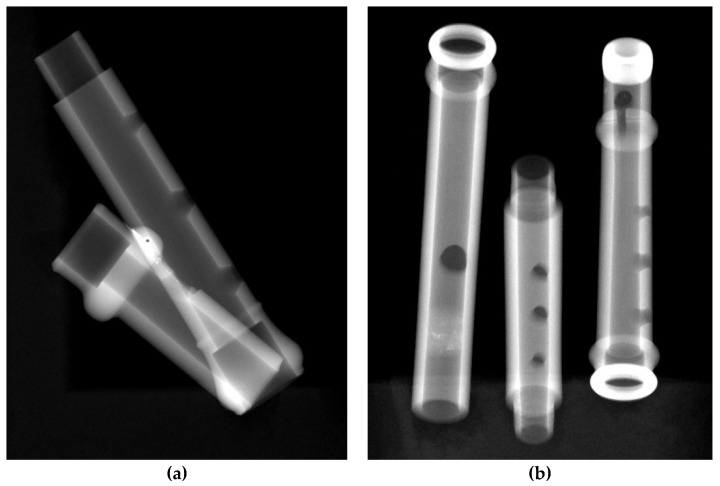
Normalized radiographs of the two analyzed instruments: (a) frontal view of the Piccolo Flute, (b) lateral view of the foot (without the brass key) and one of the two central bodies of the Transverse Flute.
